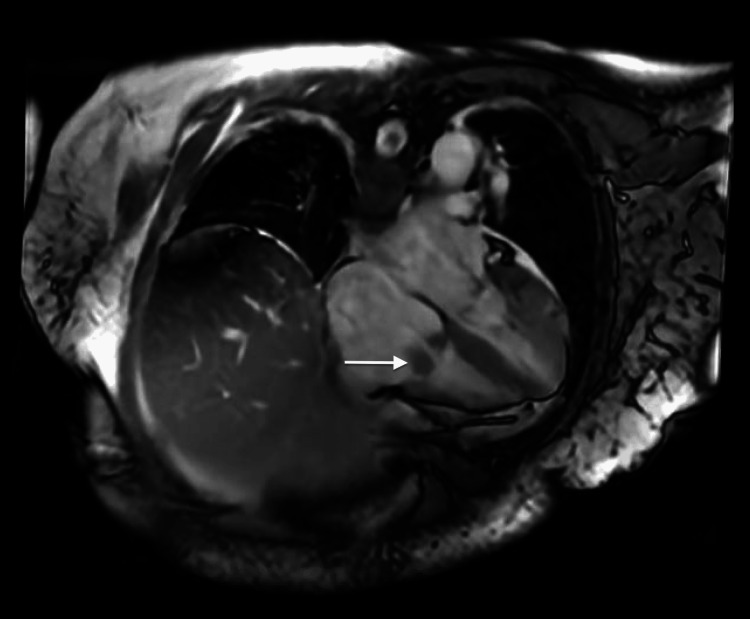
CMR showing irregular thickening of the tricuspid valve leaflets. The white arrow indicates irregular thickening of the tricuspid valve leaflets. CMR, cardiac magnetic resonance imaging
